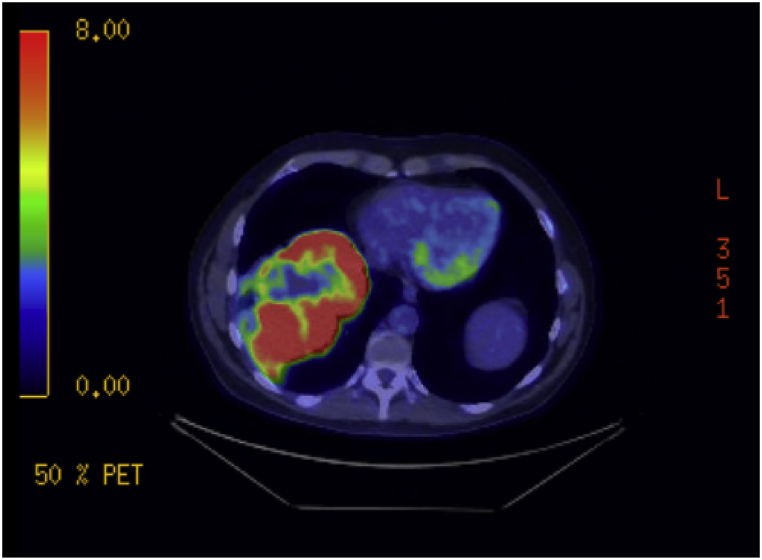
Chest positron emission tomography/computed tomography.
